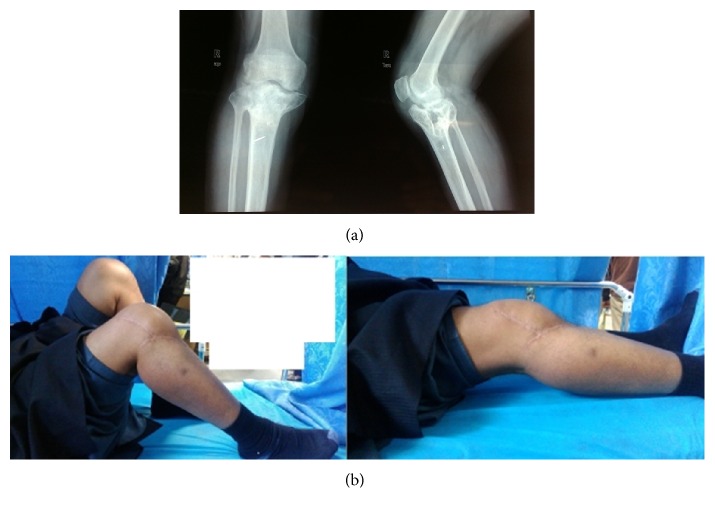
(a) Radiograph showing articular mal reduction, tibial condylar widening, and features of osteoarthritis. (b) Loss of movement at knee joint in a case who had developed deep infection.
